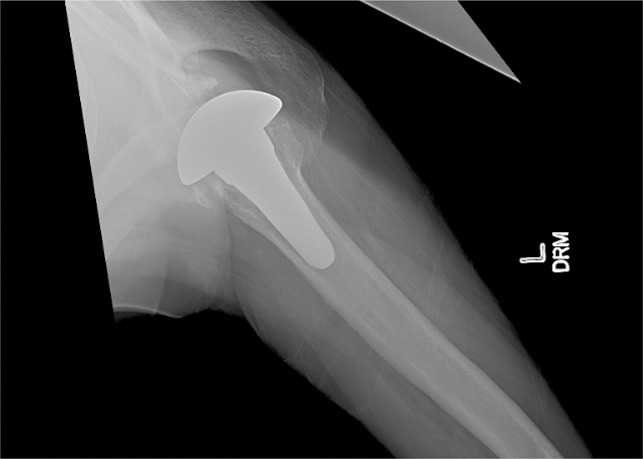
Radiograph showing the example of a displaced, nonunion of a lesser tuberosity osteotomy.
